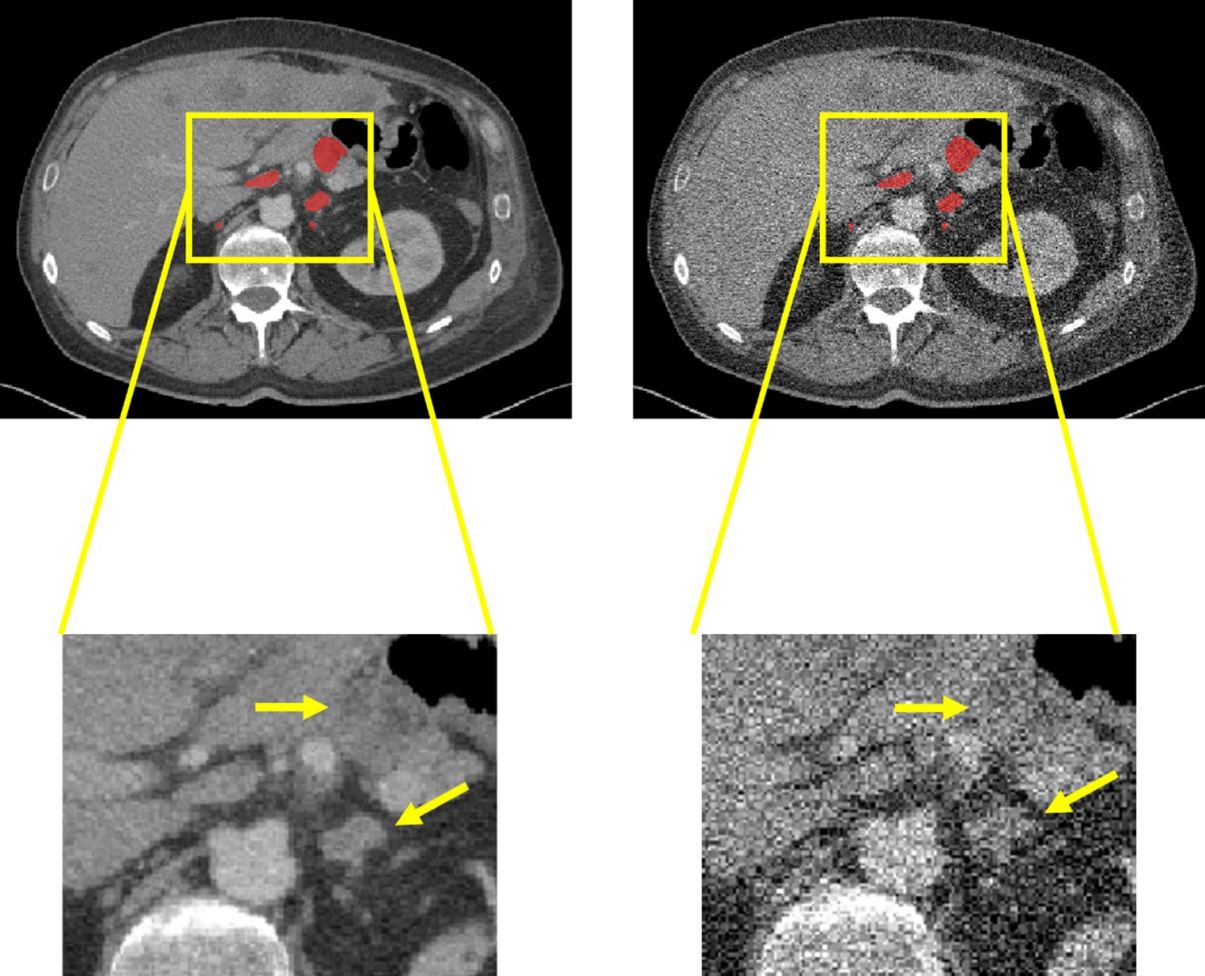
Visual example of degraded image quality and lymph node (red) visualization on a full dose CT (left) vs. 10% dose CT (right). The yellow arrows highlight two enlarged lymph nodes in the retroperitoneum of a male patient (age 61 years) with short axis diameter (SAD) of 2.3 cm and 1.7 cm, respectively. Two smaller lymph nodes are not clearly visible in the reduced dose CT
